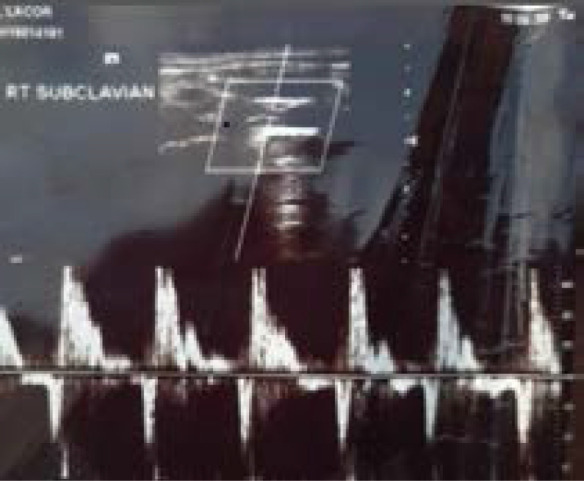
Right subclavian artery Doppler of the patient
